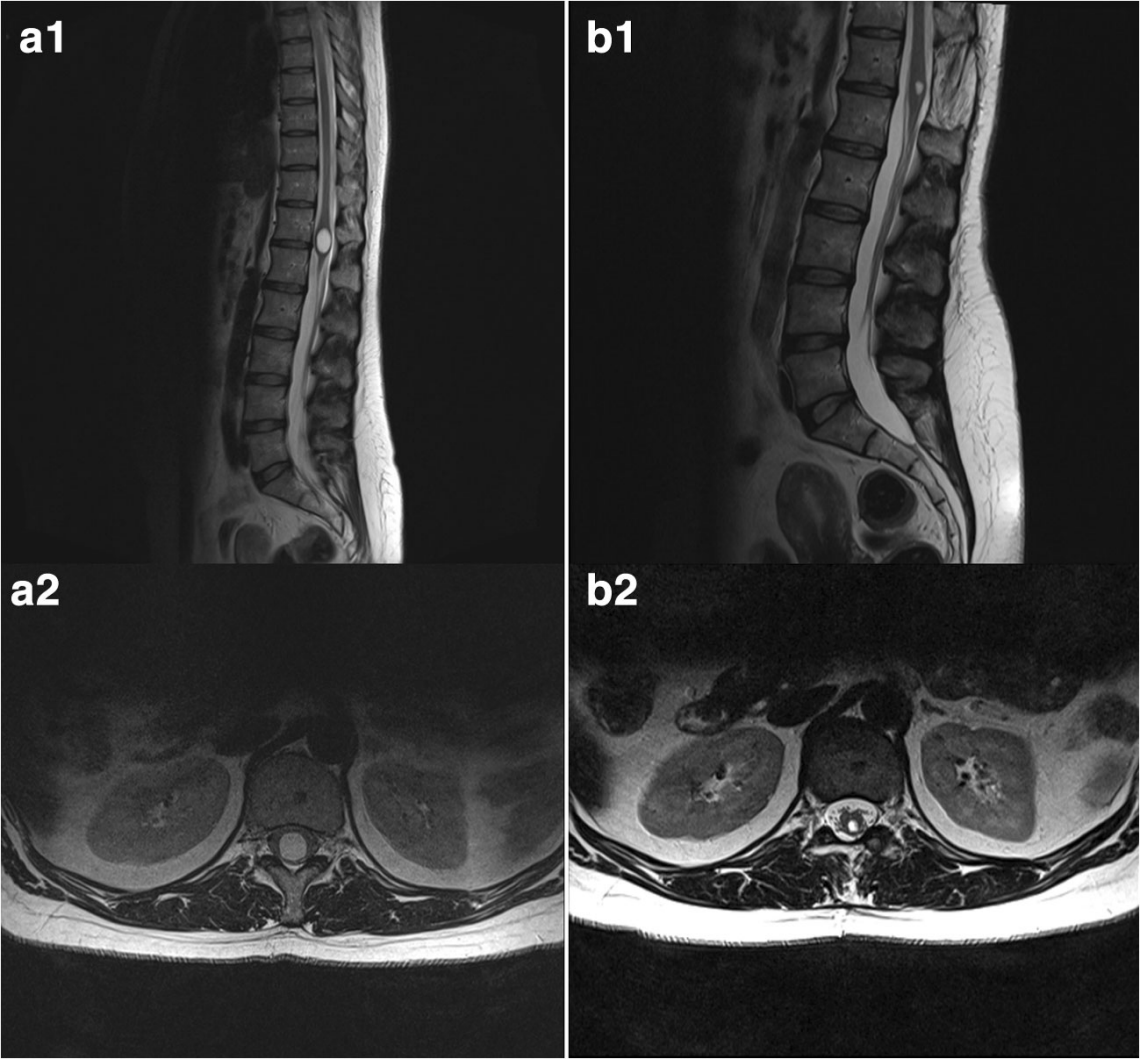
Pre-operative sagittal (A1) and axial (A2) T2-weighted magnetic resonance images showing an intramedullary ventriculus terminalis. Postoperative sagittal (B1) and axial (B2) T2-weighted magnetic resonance images showing the same lesion 3 months after cyst fenestration
